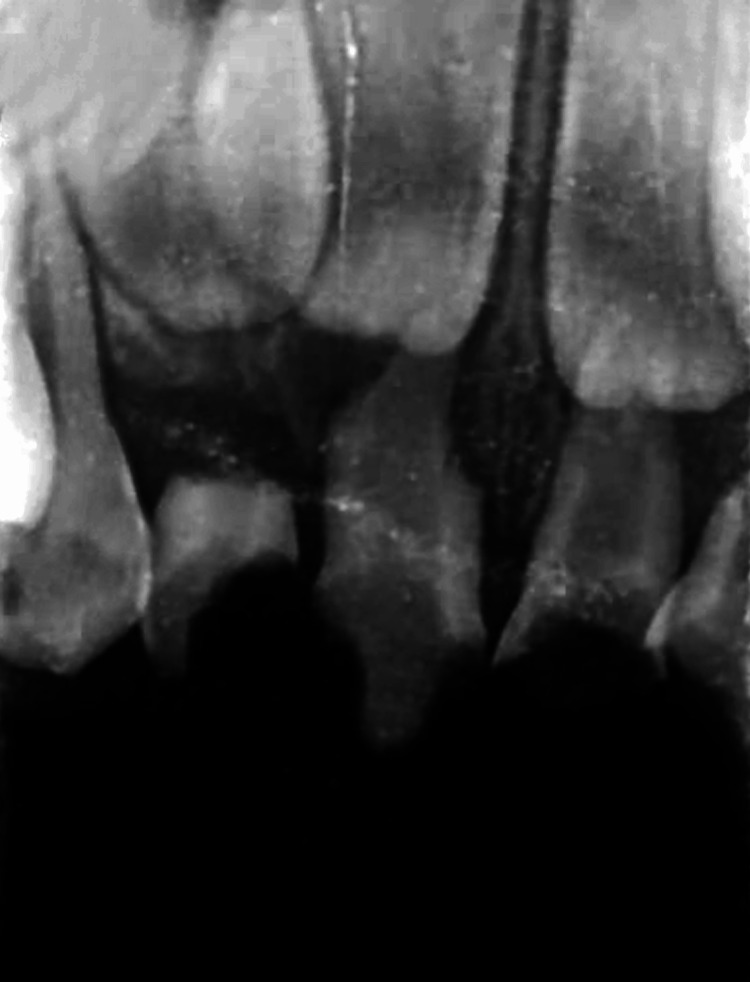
Pre-operative radiograph showing grossly decayed maxillary anterior teeth.
